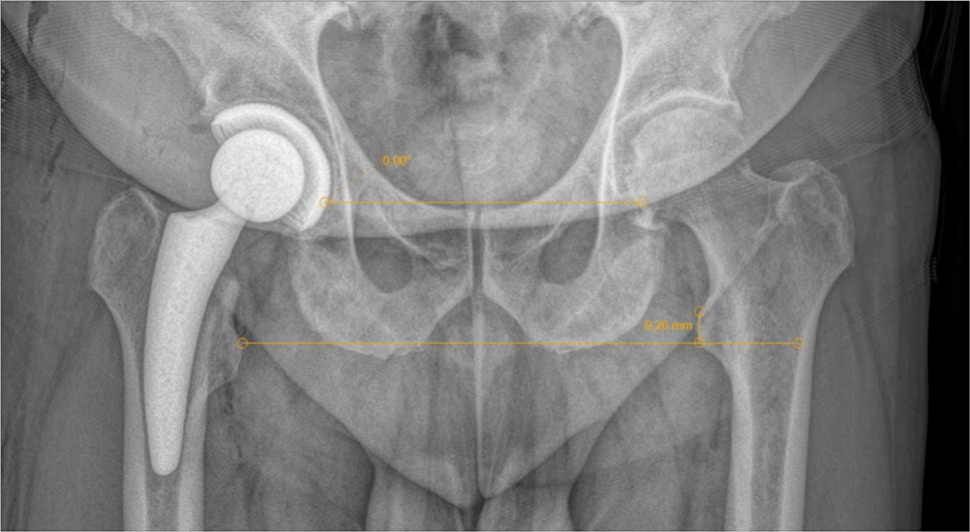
Postoperative measurement on a pelvic survey X-ray image
